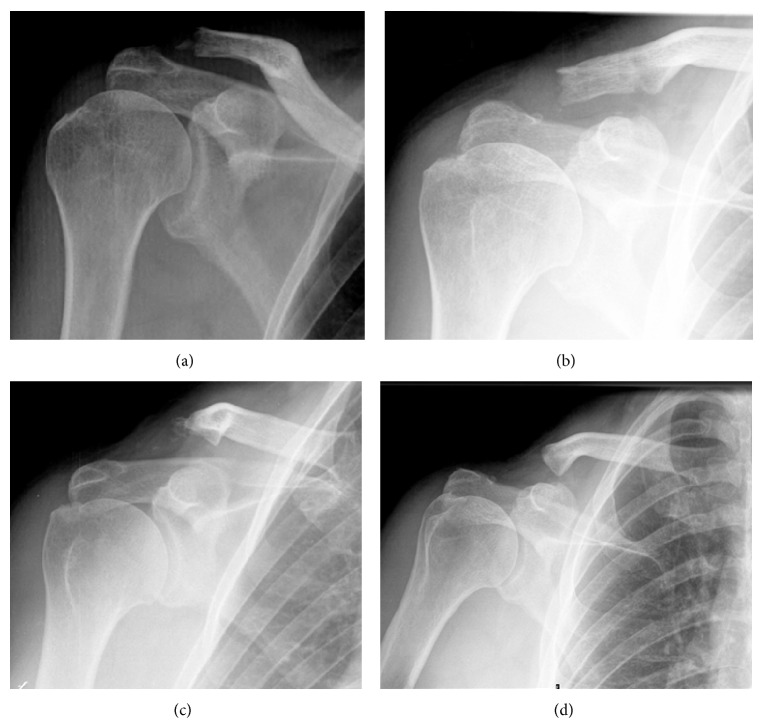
Serial of plain radiographs during the follow-up demonstrating the progression of the distal clavicle osteolysis. (a) 2 weeks after surgery. (b) 2 months after surgery. (c) 1 year after surgery. (d) 2 years after surgery.
